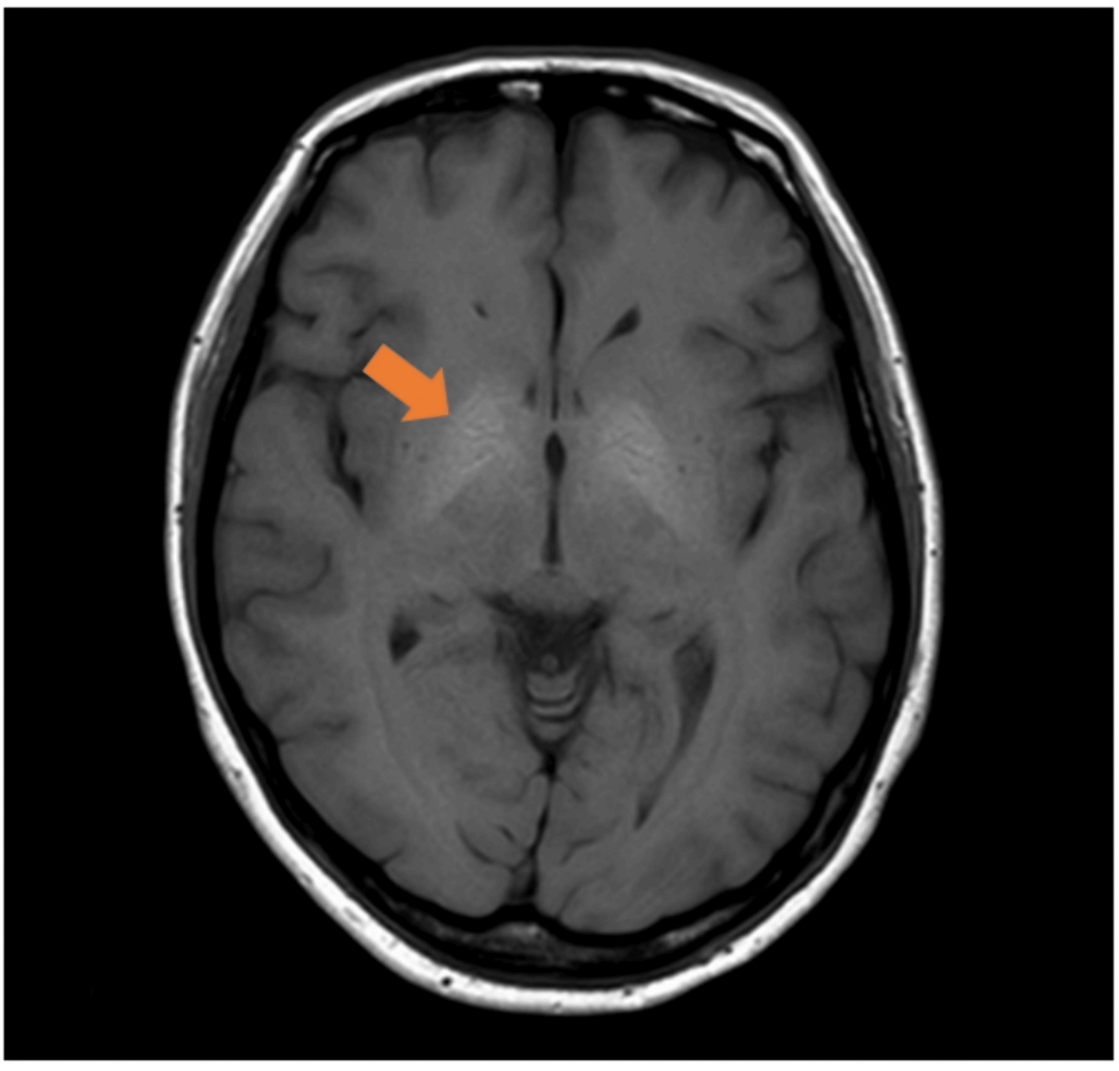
Brain magnetic resonance imaging (MRI) shows T1 hyperintense enhancement in the globus pallidus (triangle).
